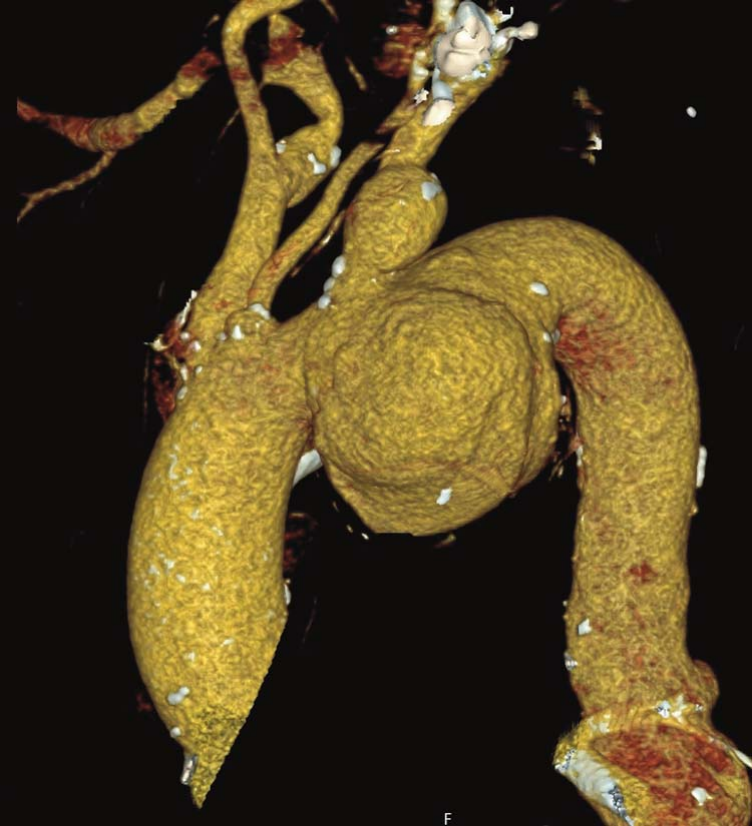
Preoperative CT reconstruction of the ascending and arch aneurysm.
